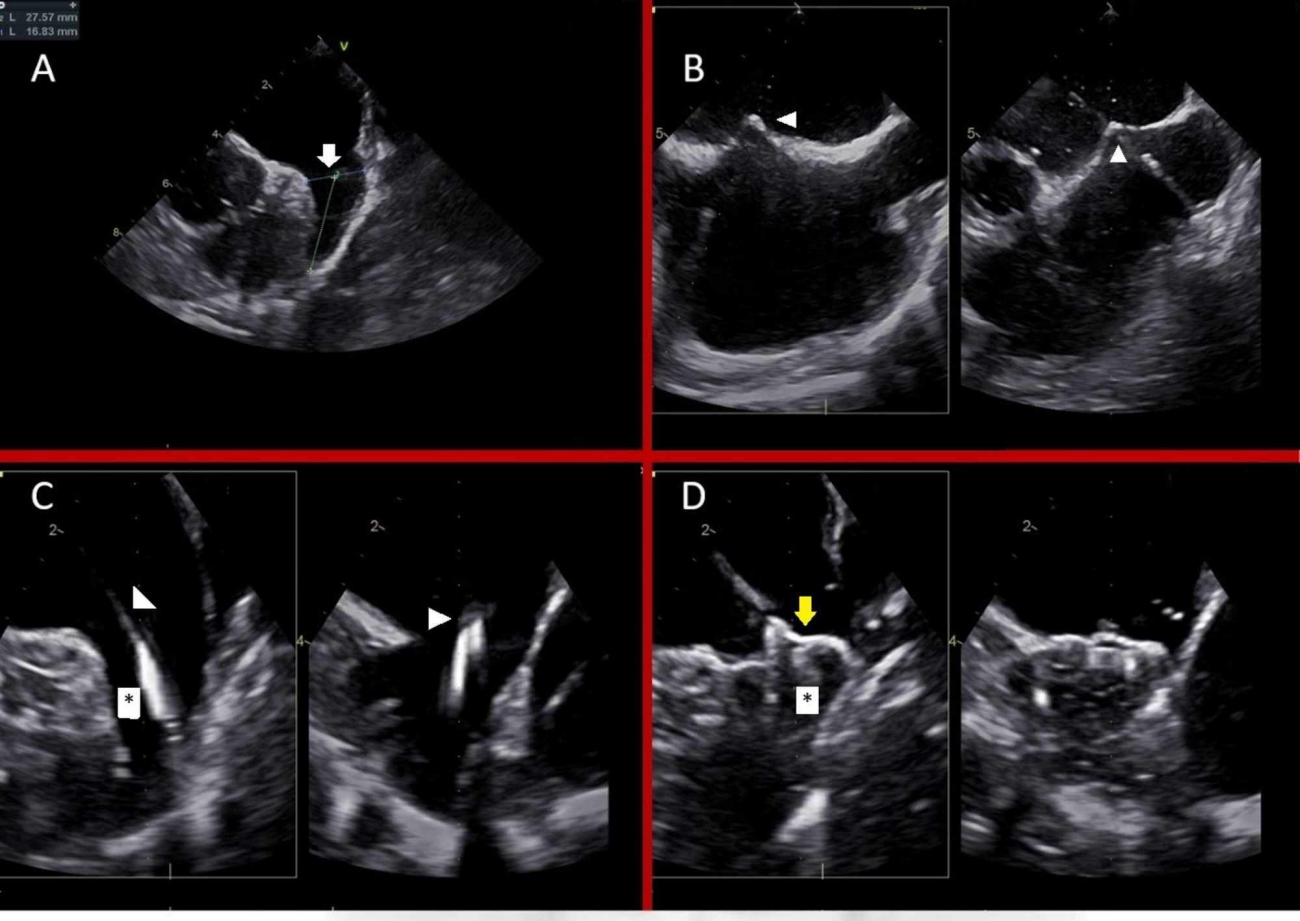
TEE-guided WATCHMAN implantation Panel A: LAA measurement on appendage view (arrow). Panel B: Bicaval view showing tenting of the interatrial septum (arrowheads). Panel C: Appendage view: Delivery catheter (arrowheads) in LAA (asterisk). Panel D: WATCHMAN (yellow arrow) implanted in LAA (asterisk). TEE: Transesophageal echocardiography; LAA: Left atrial appendage WATCHMAN: Boston Scientific, St. Paul, Minnesota
